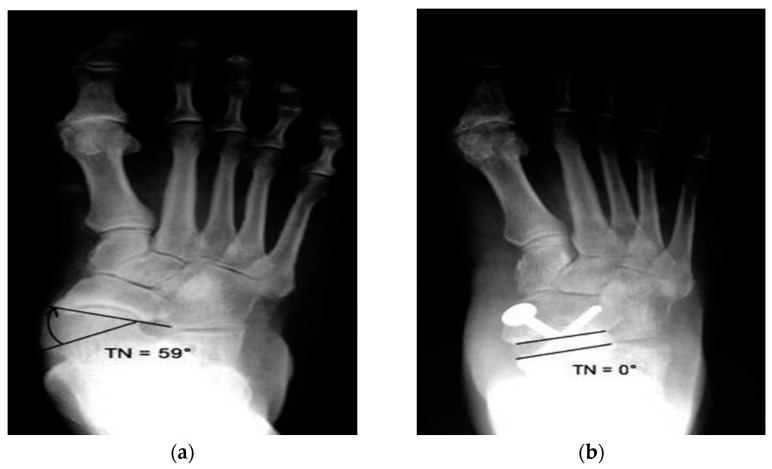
Talonavicular coverage angle measured on anteroposterior weight-bearing radiographs. Preoperative view (a) and postoperative view at final follow-up (b).
